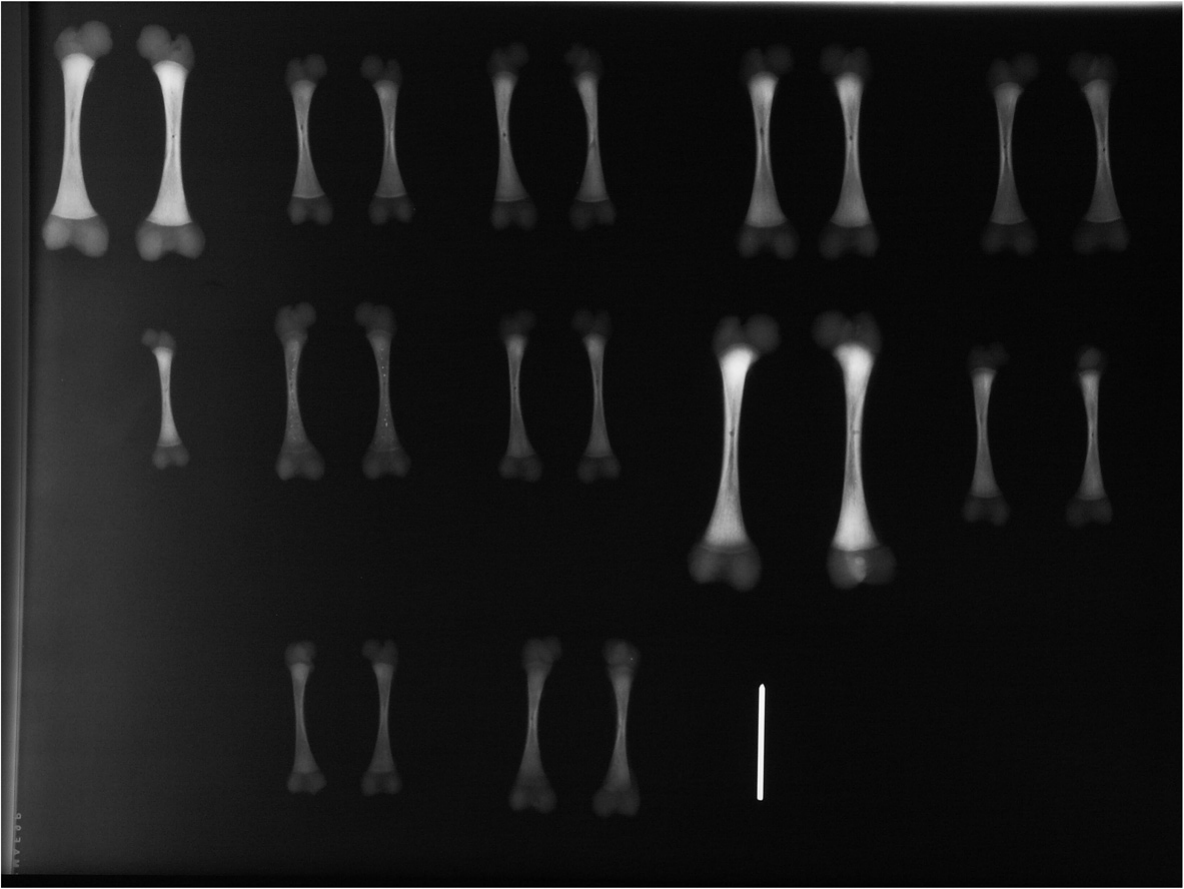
Femur radiographs were used to determine femoral shaft length (FSL) and fetal age.
